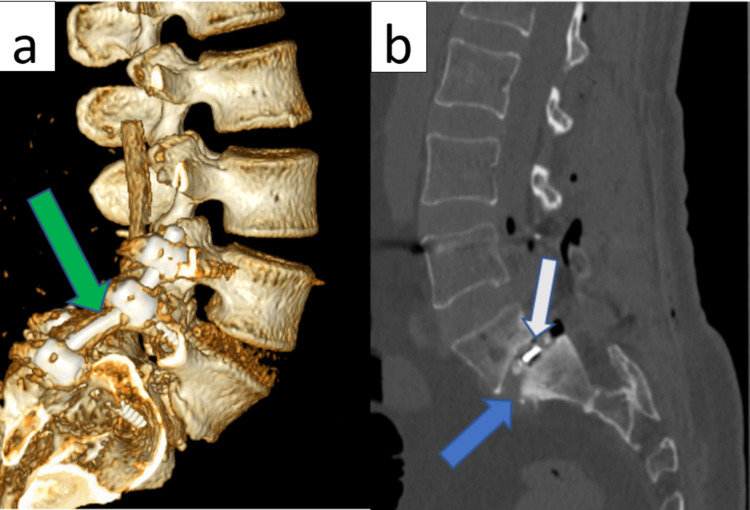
Postoperative CT control images. a: showing a CT 3D reconstruction with the six screws transpedicular fixation in L4-L5-S1 (green arrow). b: shows the L5-S1 interbody cage (white arrow) with adequate reduction of the listhesis and restoration of the height of the L5-S1 disc (blue arrow).
